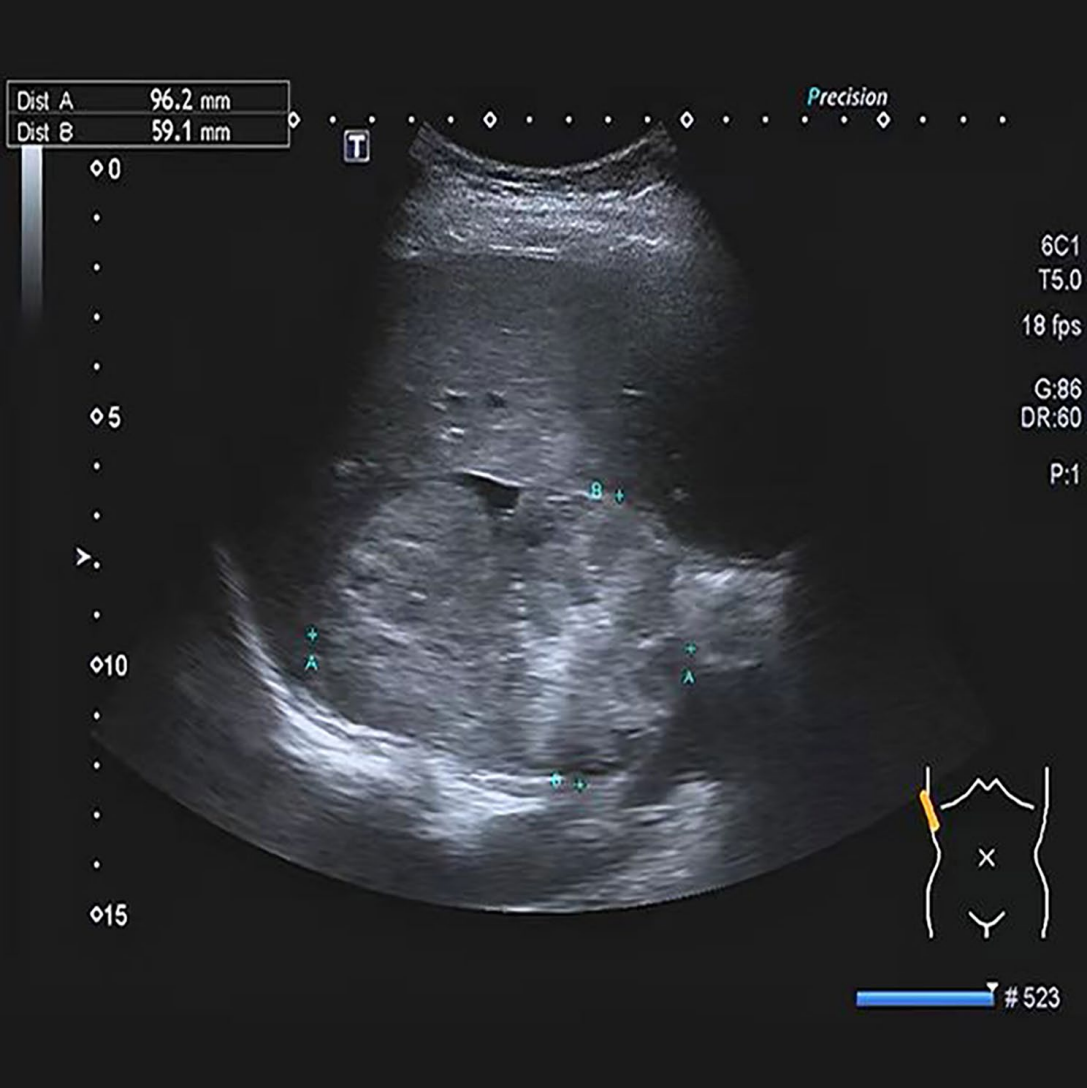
B-ultrasound of the urinary system indicated a cystic and solid space-occupying lesion in the right adrenal gland
